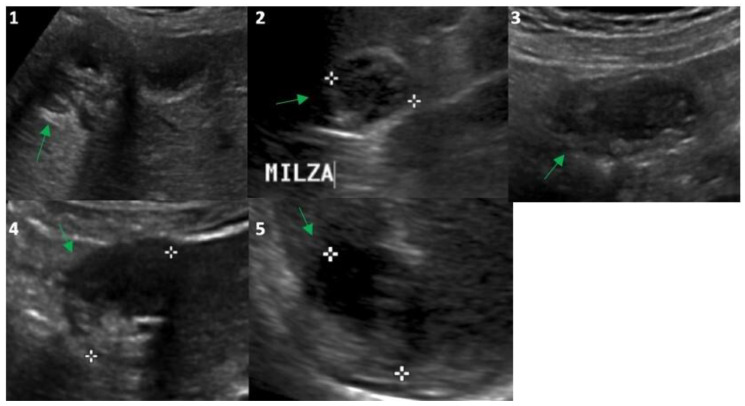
Ultrasound evaluation in 2023 of the *Echinococcus* cysts (green arrows) presented in Figure 1: segment 5 of the liver (frame (1); 25 mm), upper pole of the spleen (frame (2); 26 mm), right inframesocolic space (frame (3); 36 mm), segment 3–4 (frame (4); 30 mm) and 6 (frame (5); 30 mm) of the liver. White asterisks correspond to the points of the echo-calipers used to measure the size of the cysts.
